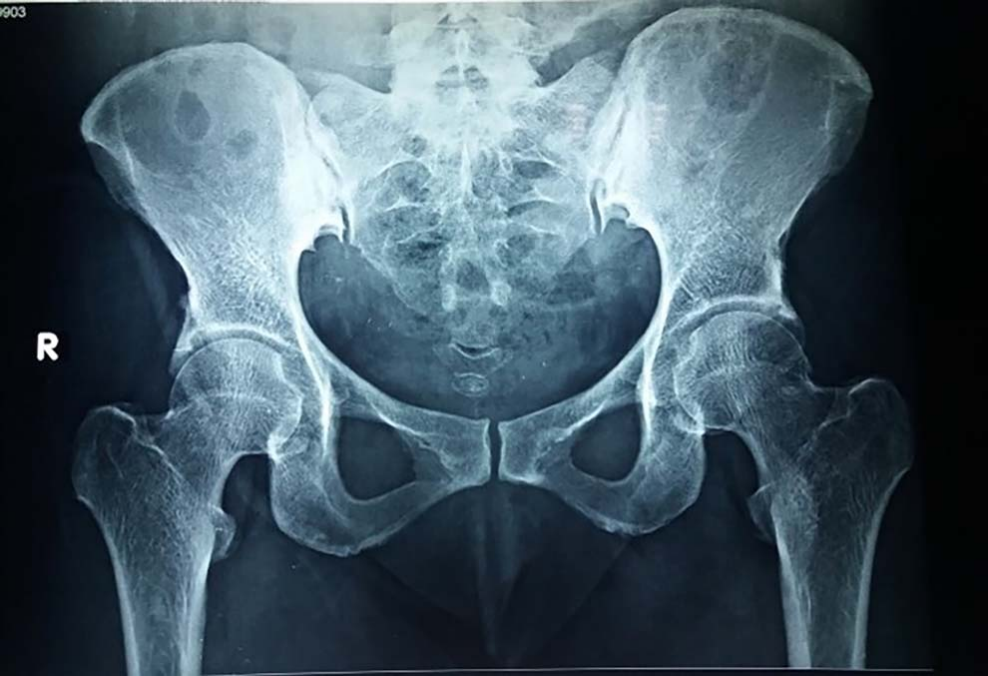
Pelvic x-ray in anteroposterior view shows ossification of the iliolumbar, sacrospinous, and sacrotuberous ligaments and the acetabular margins.
